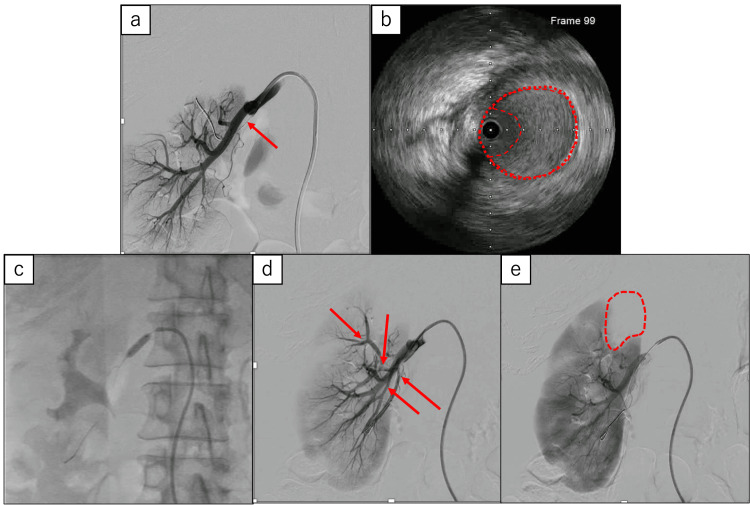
Renal angiography before revascularization reveals a complete occlusion of the ventral branch. (a) A guide wire is passed through the branch (arrow); (b) Intravascular ultrasound (IVUS) reveals the presence of extensive thrombus within the vessel lumen, and the vessel wall structure was preserved; (c) Balloon dilation of the proximal portion of the right renal artery; (d) Renal angiography following revascularization shows the four recanalized branches (red arrows); (e) Angiography demonstrates non-revascularized parenchymal regions (area enclosed by red dashed line).
